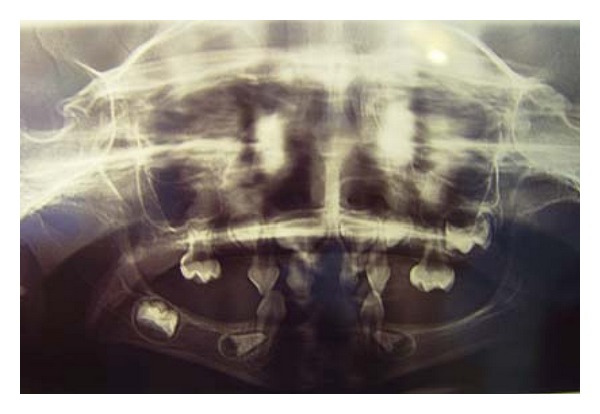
Panoramic radiograph shows eight erupted teeth of primary dentition and eight tooth buds.
